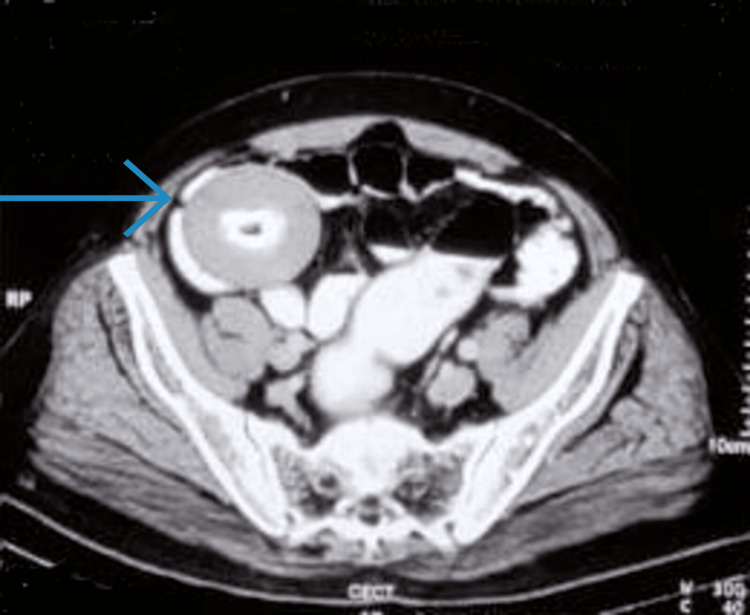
Axial contrast-enhanced CT (CECT) scan of the abdomen with IV and oral contrast demonstrating loose body, which was a hypodense center within a calcified shell surrounded by a coating of hyperdense material (blue arrow)
